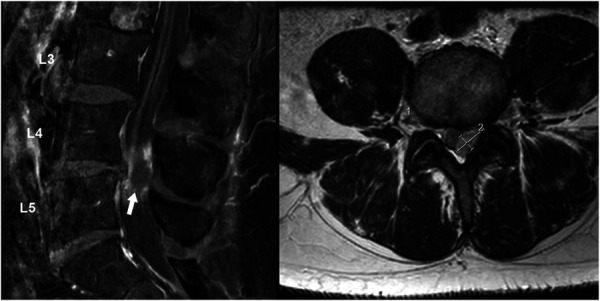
Preoperative MRI—Case 2. The sagittal T1-weighted (left) and axial T2-weighted (right) images reveal a lobulated, heterogeneously enhancing lesion of the left L4-5 cauda equina nerve roots (white arrow, left), resulting in severe canal stenosis and compression of the L5 nerve roots.
